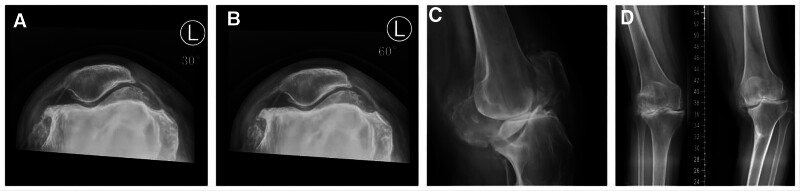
A sign of stress fracture was found in the left medial proximal tibia by X-ray examination. ((A and B) Left knee flexion = 30° and 60°. (C) Side position of the left knee joint. (D) → represents the stress fracture in the left knee joint).
